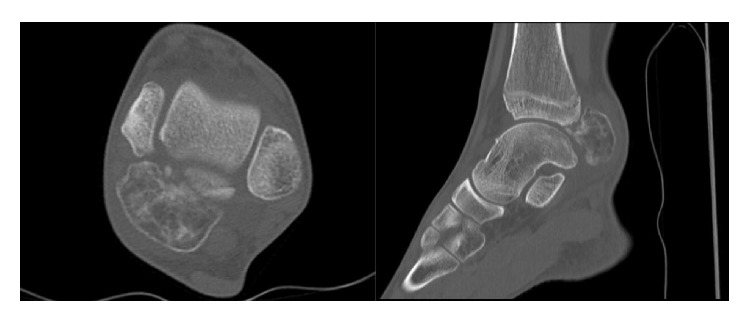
Preoperative CT images, axial and sagittal planes of the ankle joint.
